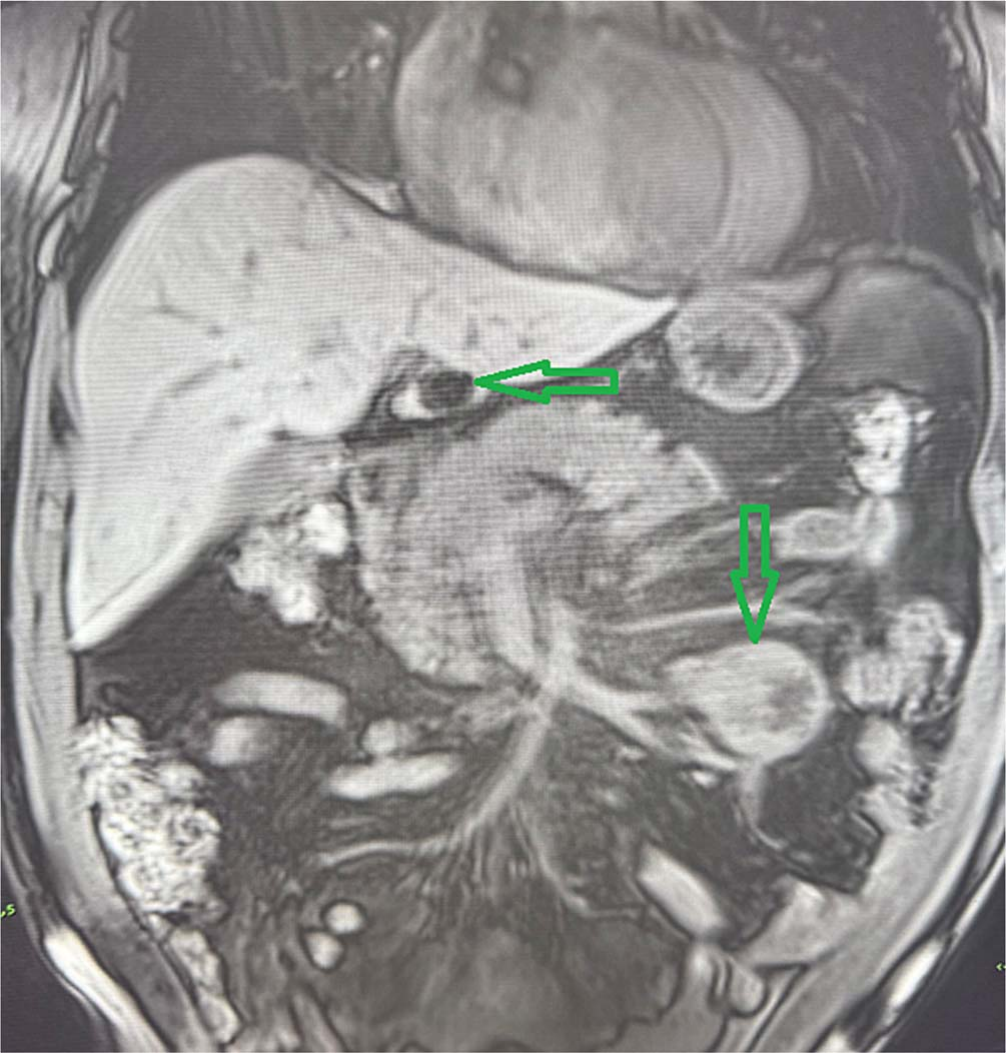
Abdominal MRI, T1 sequency. The coronal section presents a tumor mass at the root of the mesentery in the left mesogatrium and the liver cyst.
